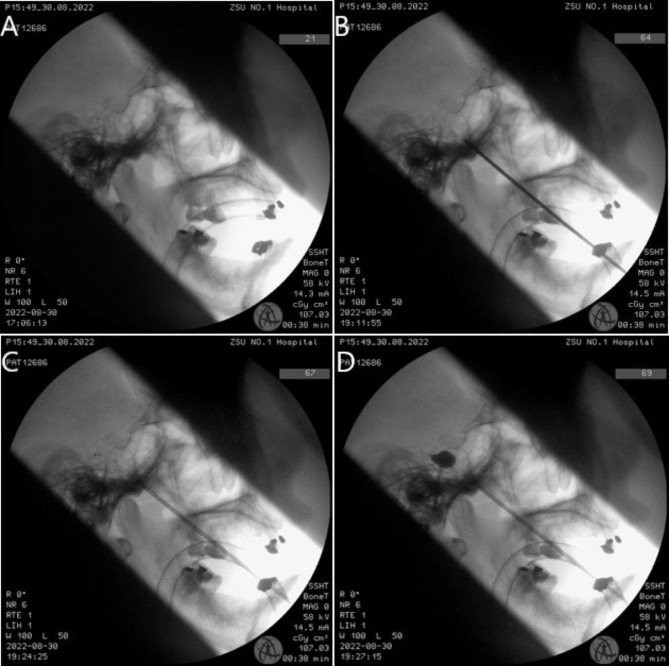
Intraoperative lateral image of the head by C-arm X-ray machine. (A) Confirmation of the standard lateral projection angle. (B) Puncture of the foramen ovale. (C) Insertation of the balloon catheter. (D) Contrast agent injection and ganglion compression
